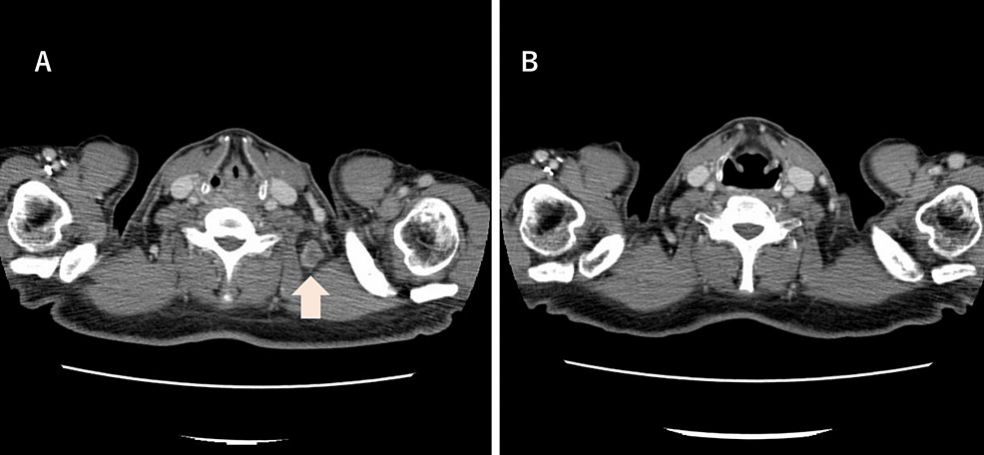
Contrast-enhanced computed tomography of the subclavicular region Contrast-enhanced computed tomography of the subclavicular region before 2nd chemoradiotherapy (A) and after the chemoradiotherapy (B). The orange arrow indicates swollen lymph nodes.
